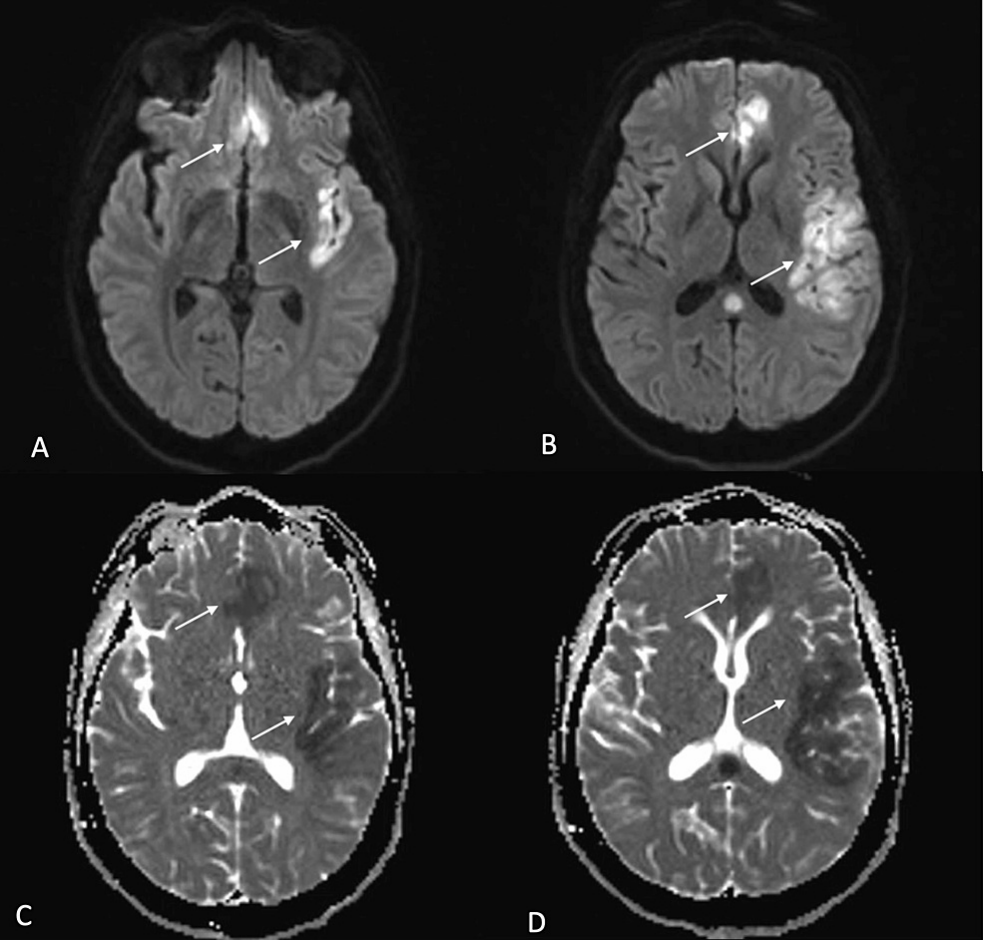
DW MRI showing diffusion restriction in the left frontal opercular cortex and precentral gyrus (A-B, white arrows), corroborated with the ADC reconstruction (B-C, white arrows) DW, diffusion-weighted; ADC, apparent diffusion coefficient
